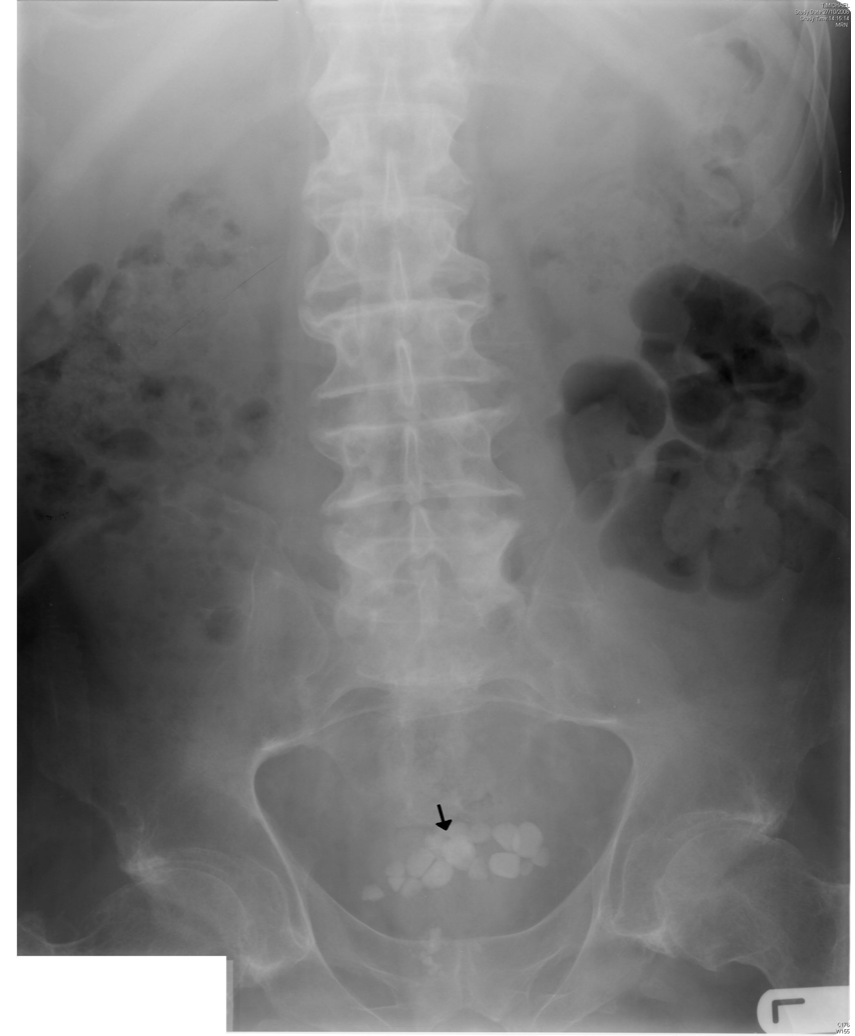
X-ray of abdomen (27 October 2006) reveals several calcified stones in urinary bladder (arrow).
